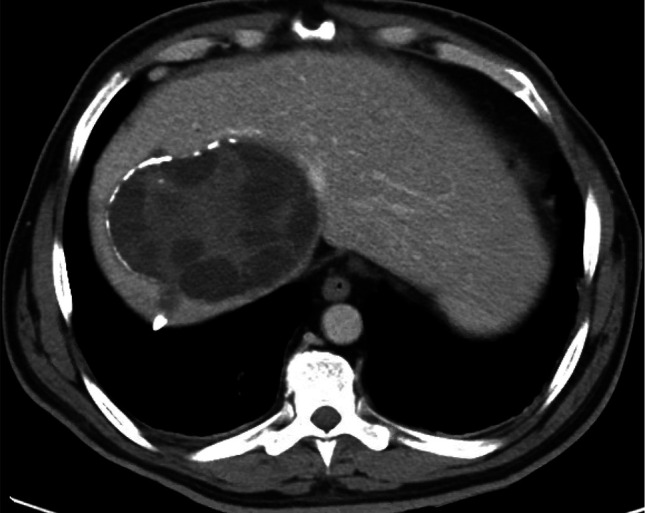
Computed tomography using a liver protocol showing hydatid cysts in the right hepatic lobe.
